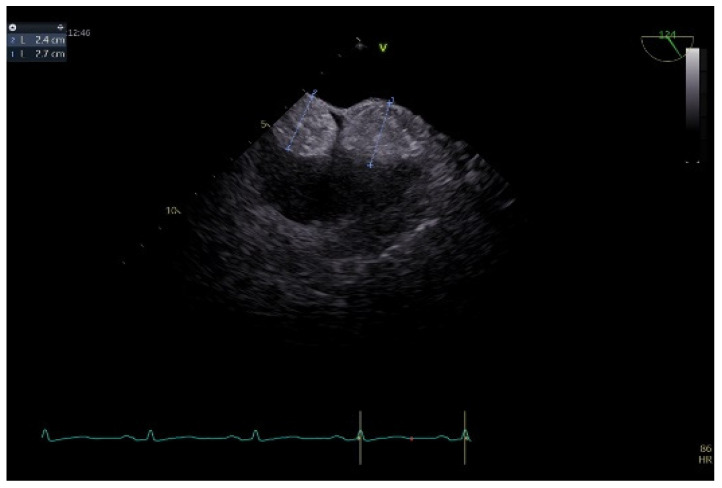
Bidimensional transesophageal echocardiography, modified bicaval view. Two so-called sessile masses (24–27 mm), at the level of the IAS respecting the fossa ovalis, with the appearance of separate structures.
